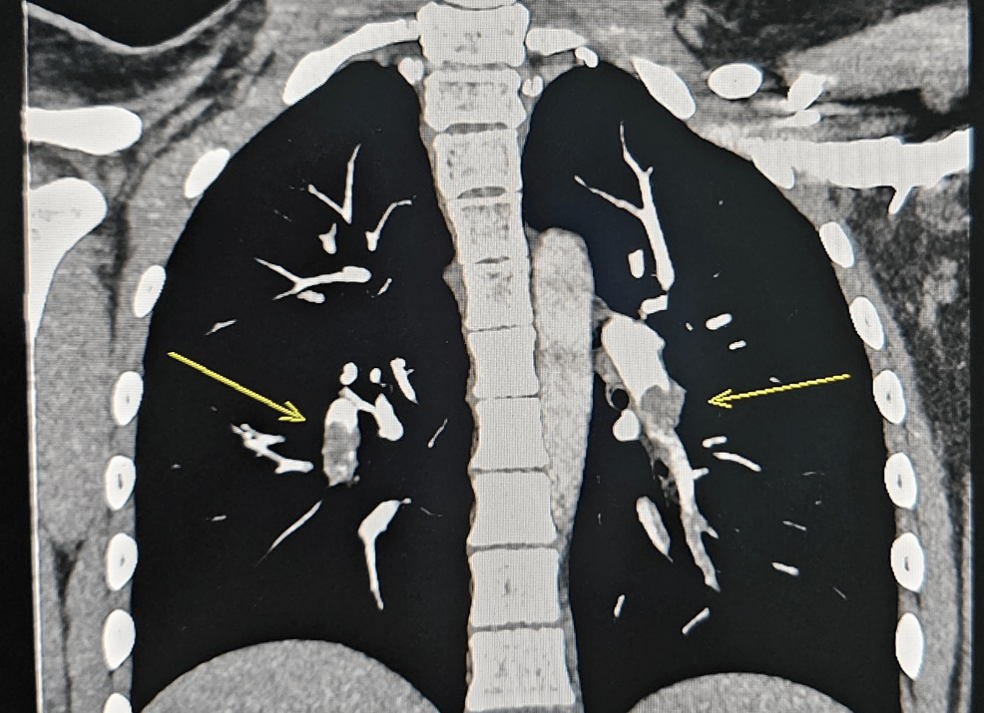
Coronal CT shows filling defects in the pulmonary artery supplying the lower lung lobe bilaterally
